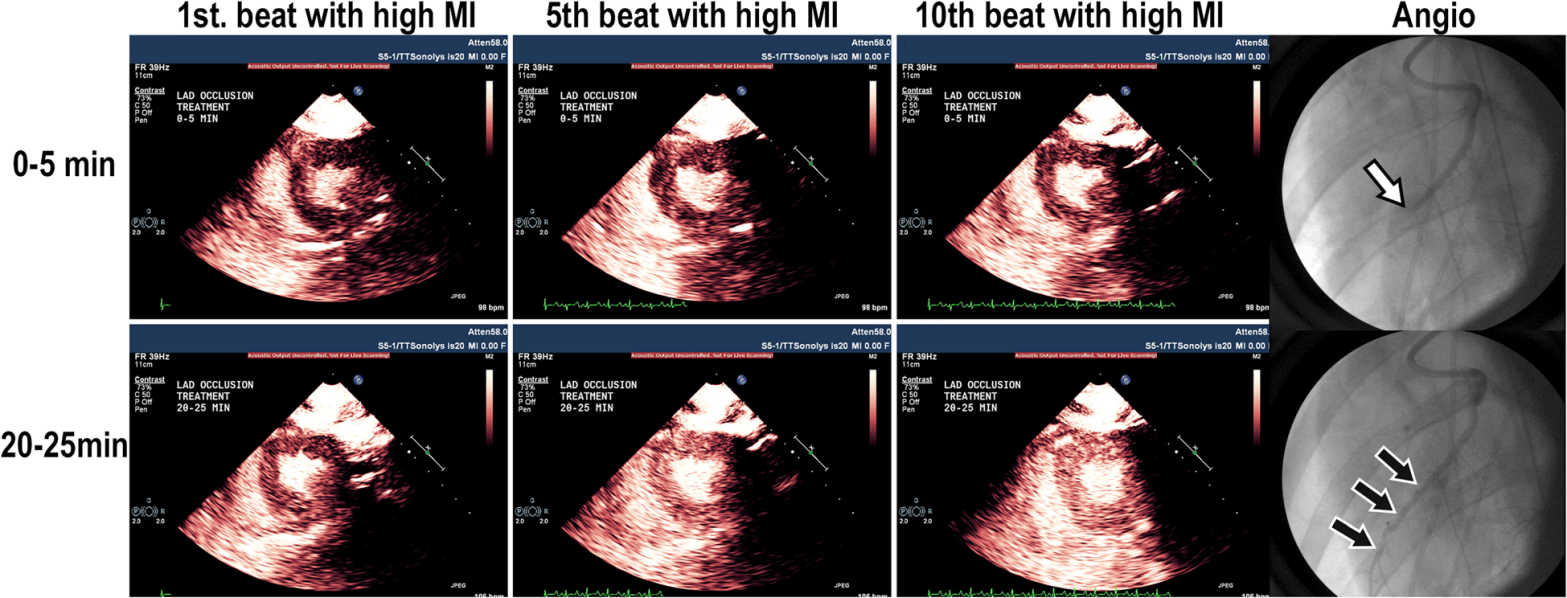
Parasternal short axis images and corresponding invasive angiograms of the left anterior descending during intermittent high MI applications of DUS while using very low MI imaging to examine the risk area in between high MI applications. At 20–25 min into therapy, the replenishment of myocardial contrast after a high MI application during the intravenous LEP microbubble infusion is more rapid and angiographic recanalization has occurred (*blue arrows*)
